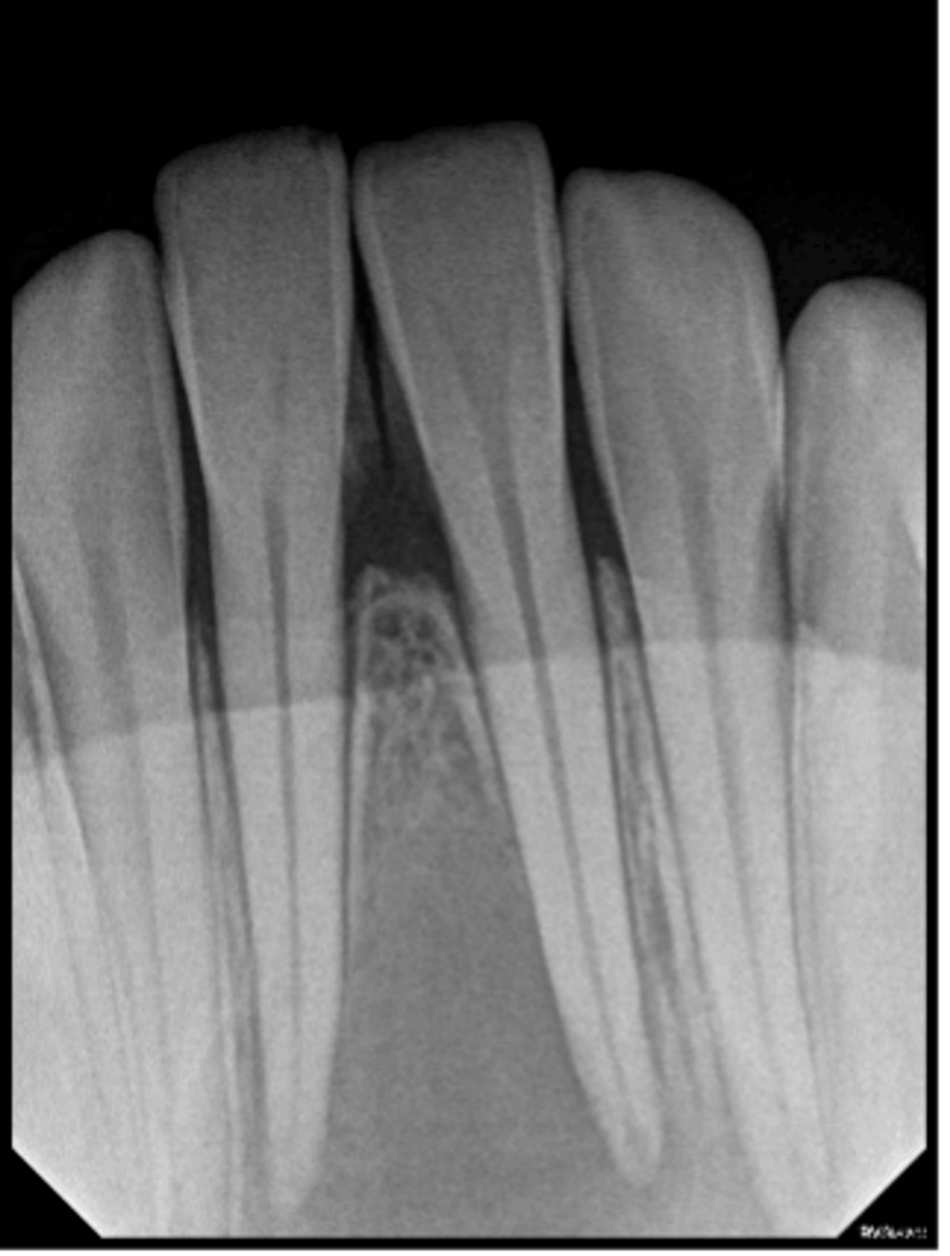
Intraoral preoperative periapical radiograph A large radiolucency was observed in the radiographic examination, extending from the left lateral incisor to the right lateral incisor, showing at the root apex and displacing the roots of both mandibular incisors.
